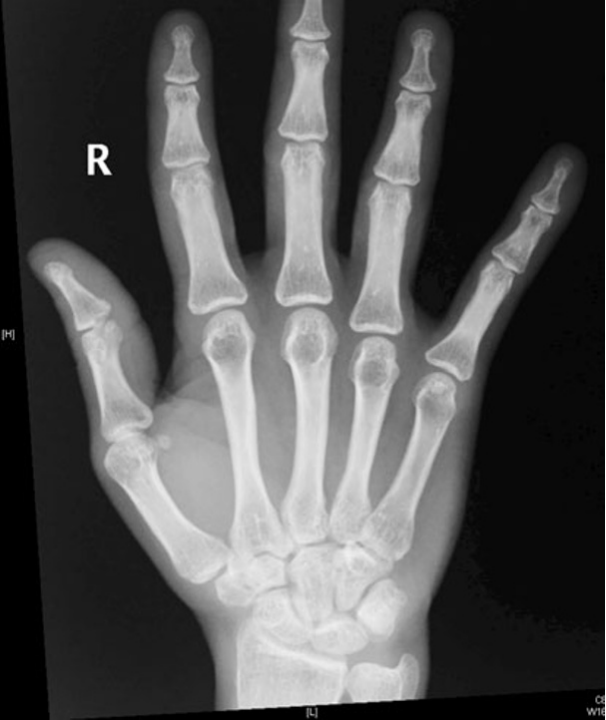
Postoperative radiograph showing complete reduction in carpometacarpal joint of right thumb
